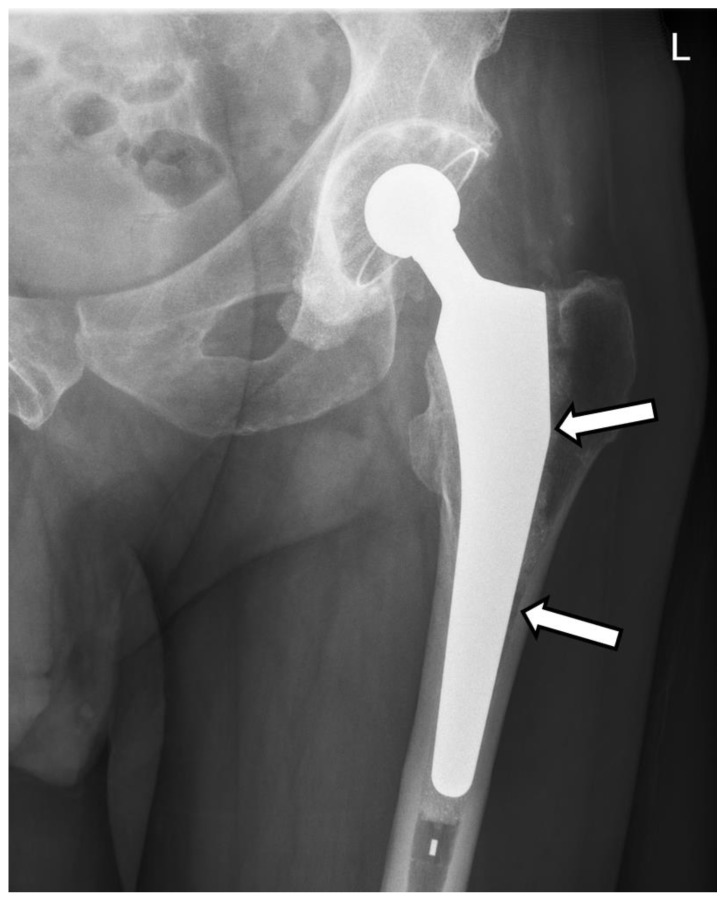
Antero-posterior X-ray of the pelvic ring and a side view of the left hip. Radiological signs of loosening of the femoral component (arrows).
